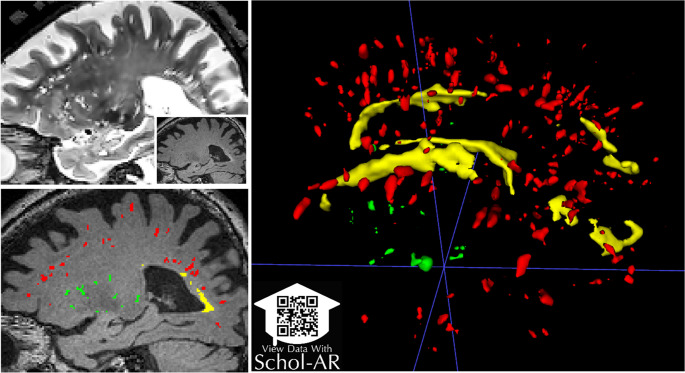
PVS segmentation from the NIFD dataset used to test the T2w+FLAIR model. Top-left: Raw T2w scan with corresponding FLAIR scan (inset). Bottom-left: PVS segmentation generated by the T2w+FLAIR nnU-Net overlaid onto the corresponding FLAIR scan with WM-PVS (red), BG-PVS (green), and white matter hyperintensities (yellow). Right: 3D render of the PVS segmentation augmented with Schol-AR and viewable via the QR code [35]
